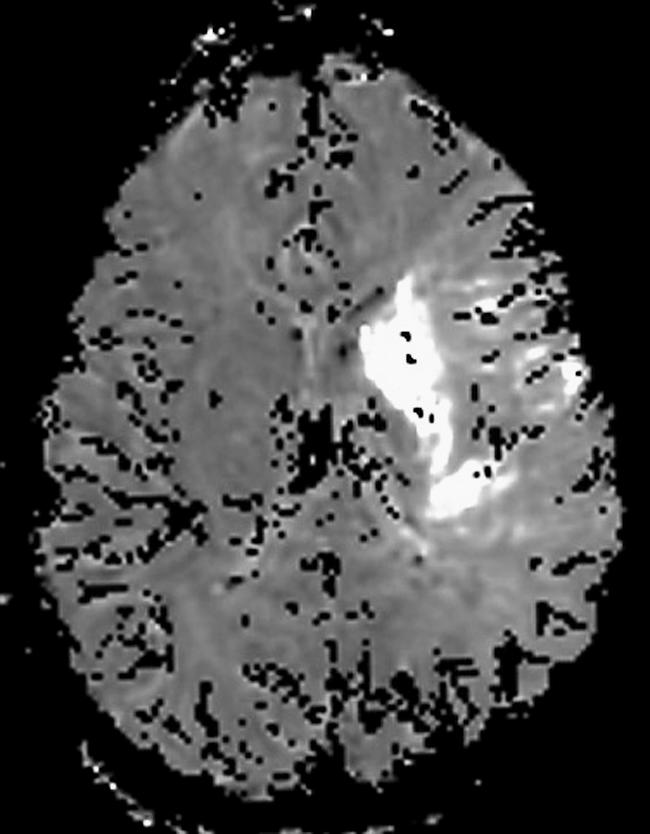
Diffusion-Weighted Imaging (DWI) of the Brain Diffusion defect indicating irreversible left hemispheric ischemic damage in the area controlling right hand and leg function.
